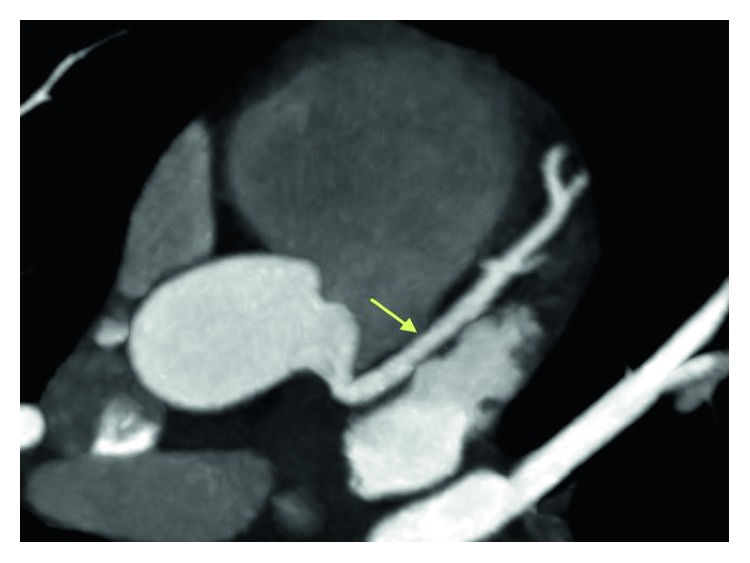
Coronary CT angiogram image revealing normally positioned left anterior descending artery (LAD) without any evidence of coronary artery disease.
